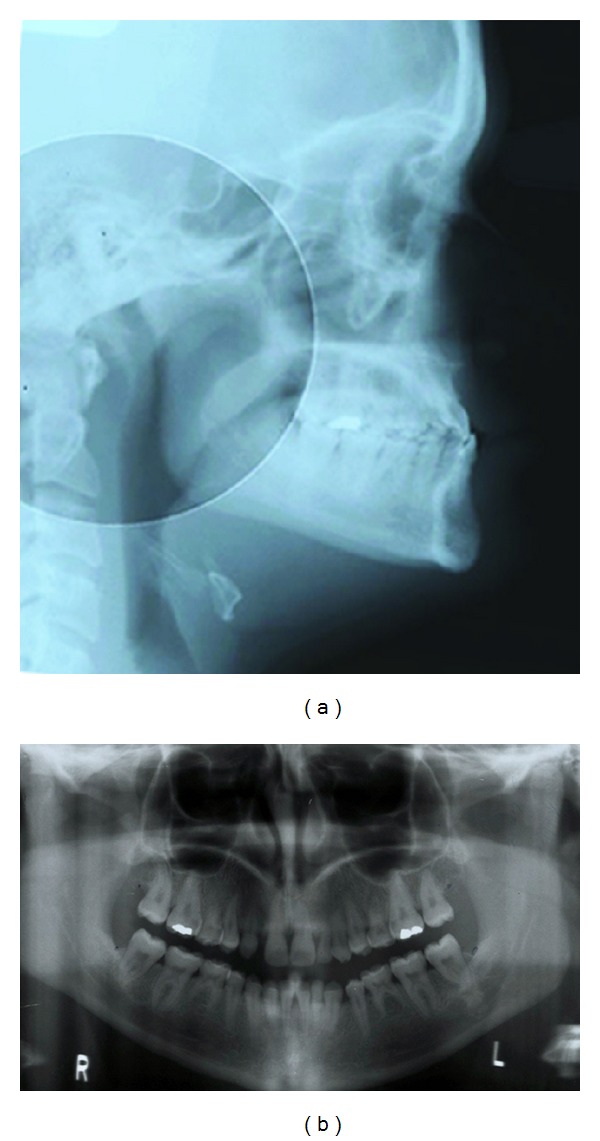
(a,b) Lateral cephalometric and panoramic radiographs of an adolescent with Down syndrome show typical skeletal disharmony, malocclusion, and permanent tooth agenesis.
